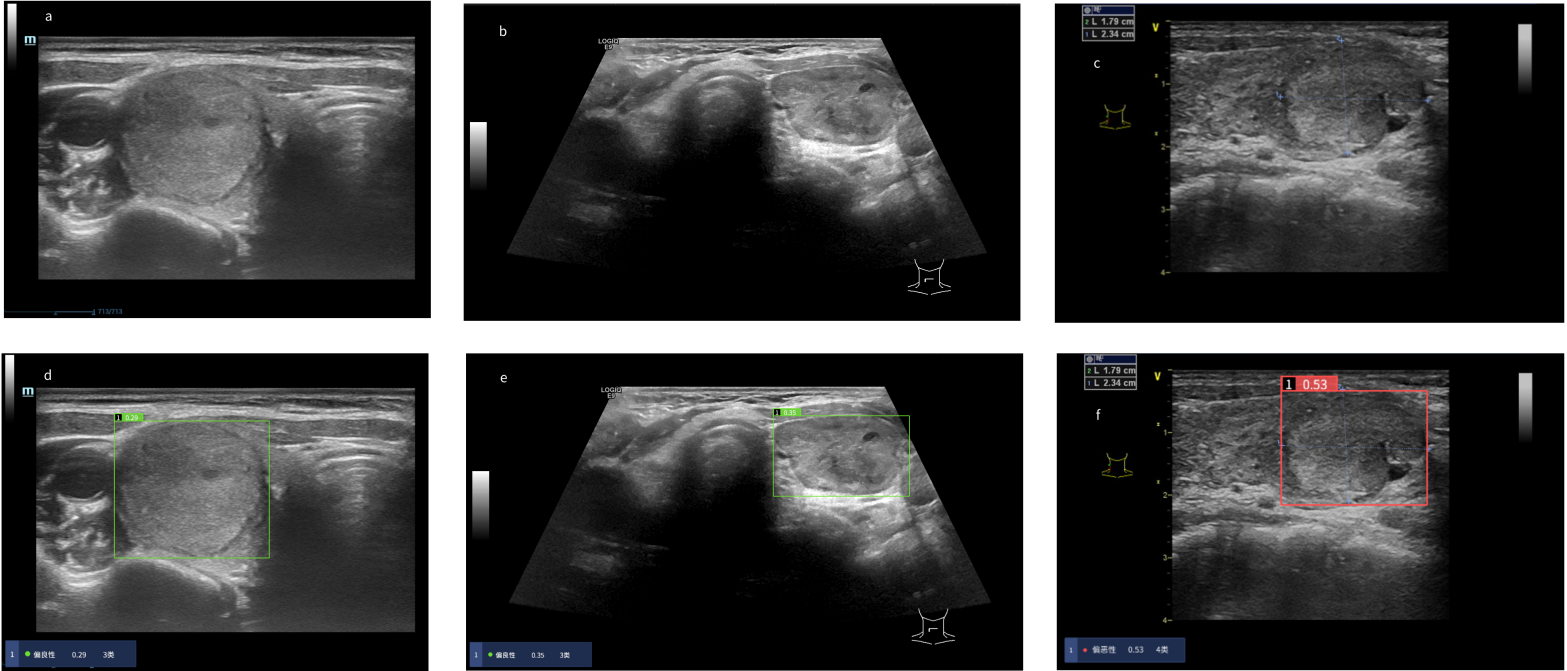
Risk coefficient assessment and diagnosis of thyroid nodules in the AI system. When the risk coefficient of thyroid nodules was < 0.4, the AI system diagnosed the thyroid nodules as “benign” as noted in the green display box, otherwise “malignant” as noted in the red display box. (A–C) Original ultrasound images of thyroid nodules. (A) Pathological diagnosis: thyroid follicular adenoma. (B) Pathological diagnosis: adenomatoid hyperplasia nodule. (C) Pathological diagnosis: follicular thyroid carcinoma. (D–F) Diagnosis of thyroid nodules in the AI system. (D) The AI system diagnosed the nodule as “benign”. Three radiologists diagnosed the nodule as “malignant”. (E) The AI system diagnosed the nodule as “benign”. Three radiologists diagnosed the nodule as “malignant”. (F) The AI system diagnosed the nodule as “malignant”. Three radiologists diagnosed the nodule as “benign”.
